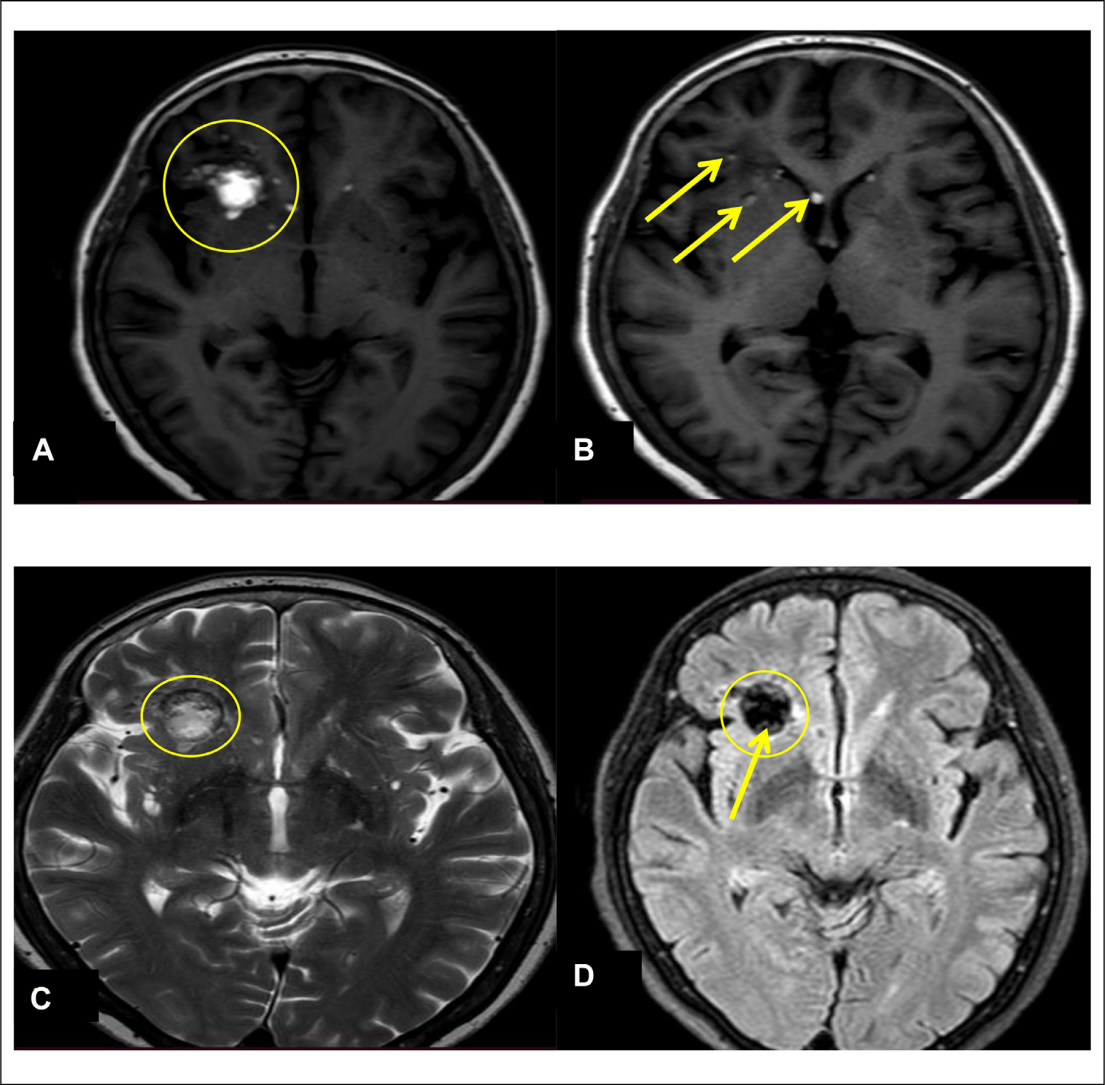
MRI brain (T1 weighted axial MR sequence) showing a well-defined, round to ovoid lesion measuring approximately 19×18×24mm (APxTRxCC) in right basisphenoid appearing intensely hyperintense (marked by circle). (B) Few disseminated hyperintense droplets in adjacent brain parenchyma, sulcal spaces and in frontal horns of bilateral lateral ventricles (marked by arrows). (C) Heterogeneously hyperintense lesion in right basisphenoid region was revealed in T2-weighted sequence (marked by circle). (D) Heterogeneously hypointense lesion noted in FLAIR image representing fatty components (marked by arrow).
